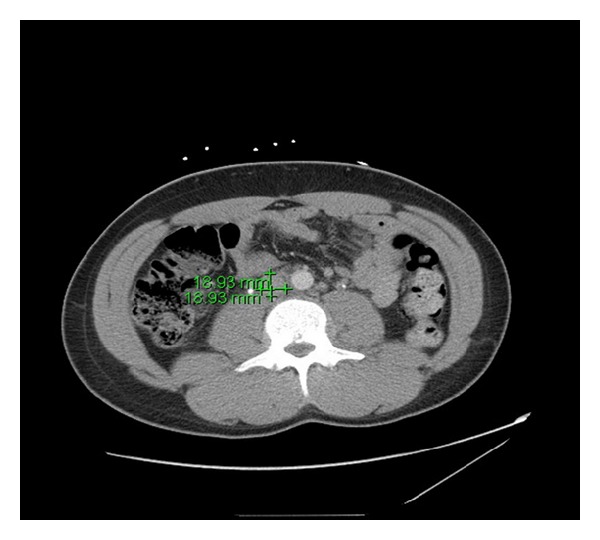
Dilated abdominal veins and IVC thrombosis visible on computed tomography.
